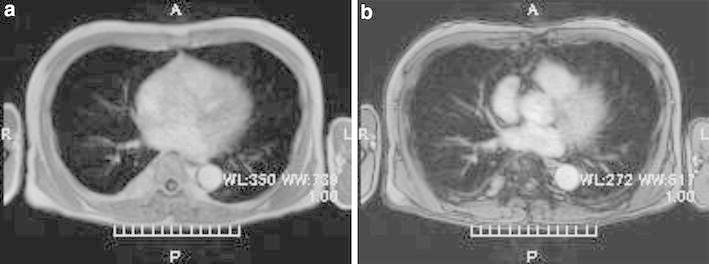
Magnetic resonance imaging (MRI). According to T1 enhanced dual image at (TR165, TE4.8), the tumor is revealed as high intensity (Fig. 3a), however, at (TR165, TE2.3) it is revealed as partially low intensity (Fig. 3b). It suggests that the tumor contains fat component
